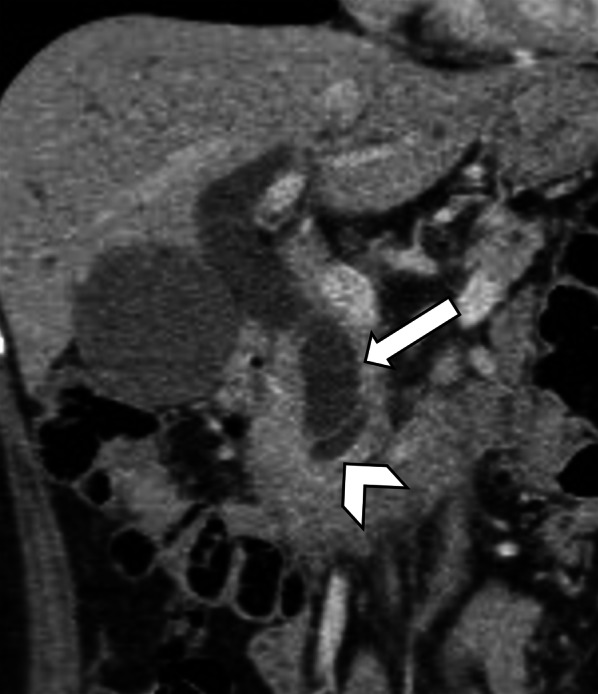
Metastatic ILC of the pancreas in a 53-year-old female presenting with painless jaundice. She was on adjuvant letrozole for Stage 2 ILC of the left breast diagnosed 2 years ago. Coronal contrast-enhanced CT image shows a dilated common bile duct (white arrow) and dilated pancreatic duct (arrowhead)—the double duct sign. The intrahepatic ducts are also mildly dilated. No discrete mass is seen in the pancreatic head on CT or on EUS. Metastatic involvement of the pancreatic head was confirmed on intra-operative biopsy
